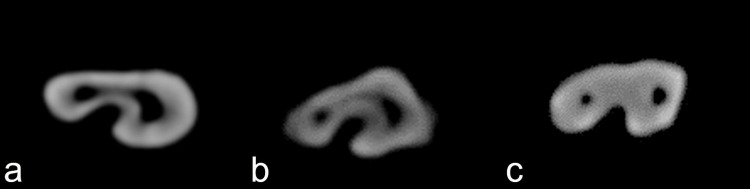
Cone beam computed tomographic images (axial section) of the types of C-shaped canal configurations according to fan’s classification found in the present study a) Type C1, b) Type C2, and, c) Type C3
